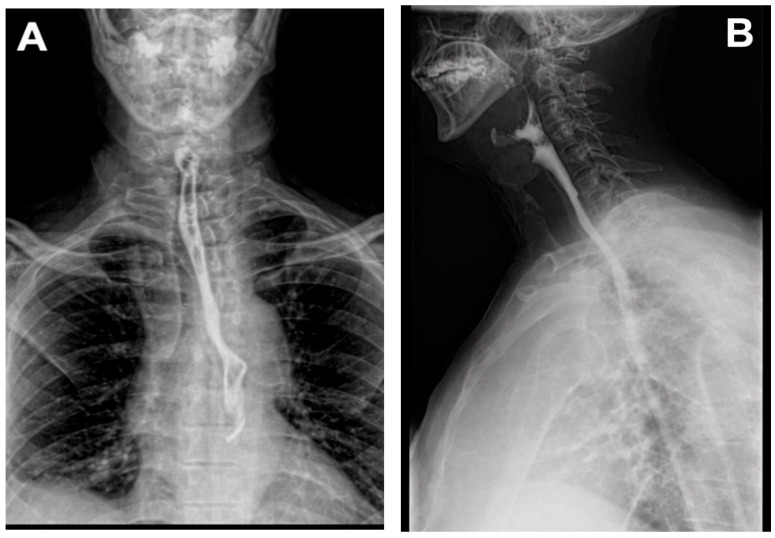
(A,B) Barium swallow test radiography showing continuous flow through the esophagus.
